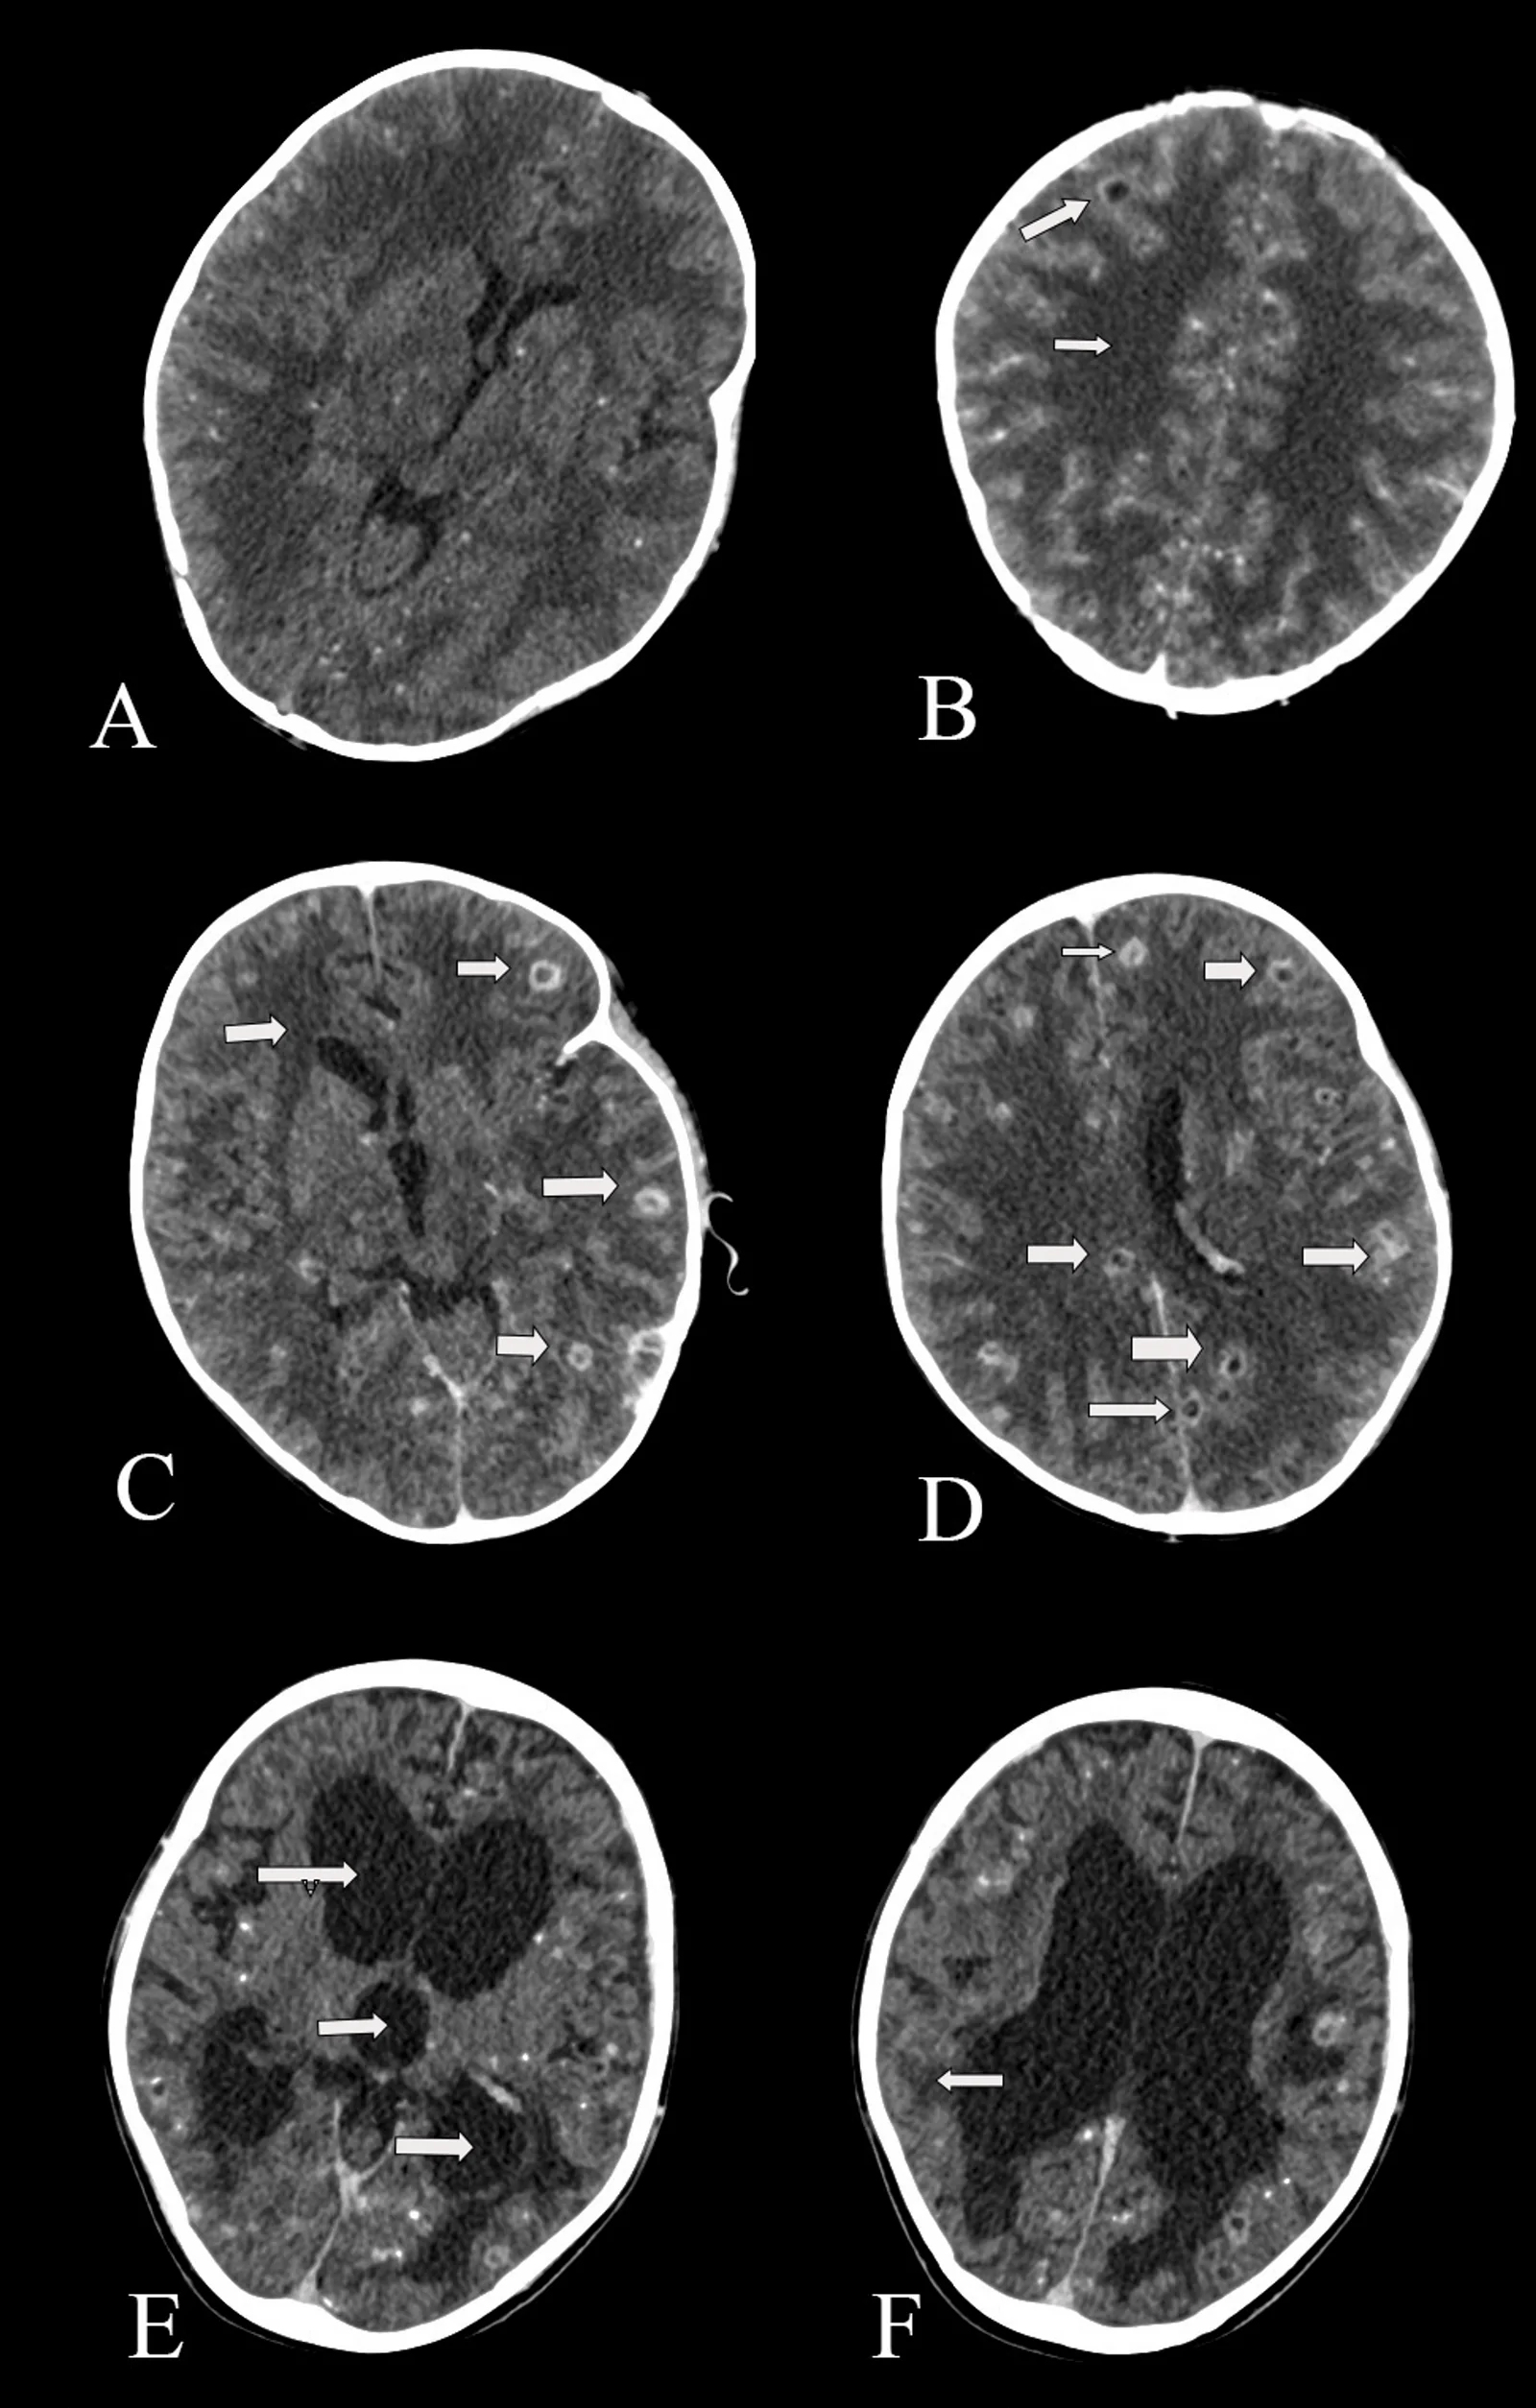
Brain CT scan of a seven-year-old boy with massive ring-enhancing neurocysticercosis lesions and extensive edema after albendazole treatment (A, B); additional ring-enhancing lesions appearing six months later (C, D); and the final evolutionary stage with calcifications, cortical and subcortical atrophy, and ventricular enlargement (E, F)
